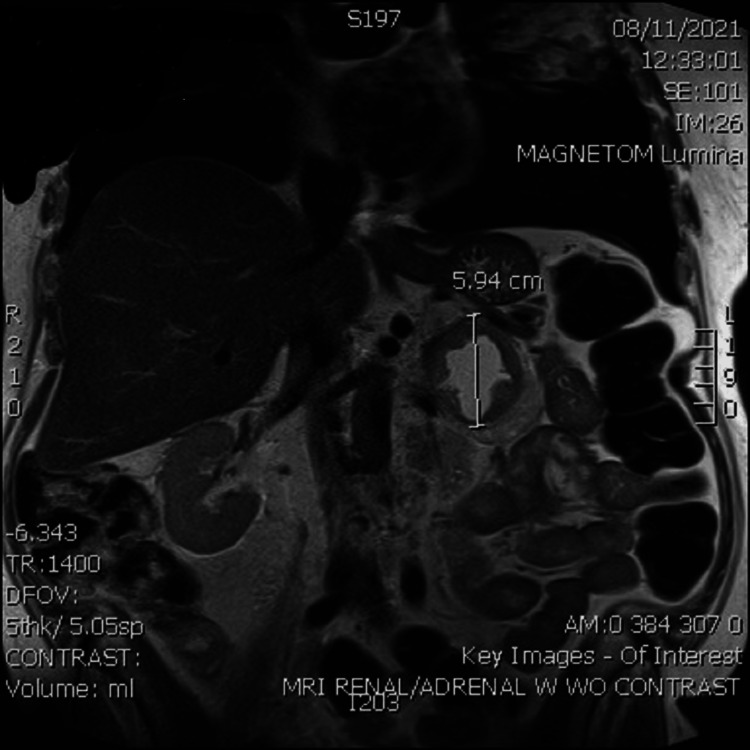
Magnetic resonance imaging showing the frontal view of the abdomen. There is a 5.94 cm mass in the left adrenal gland. There is a thickened rim of soft tissue hyperenhancement surrounding the mass.
